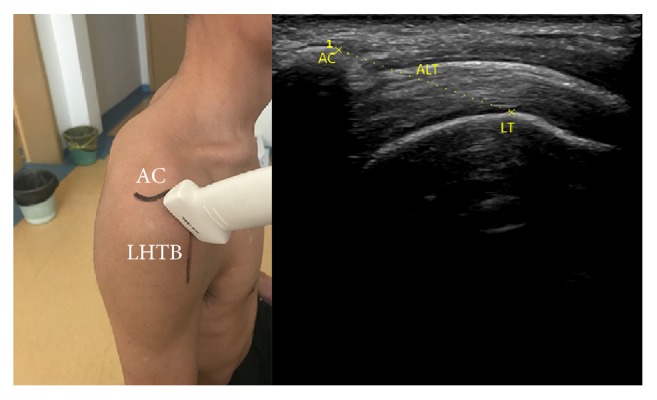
Anatomical structures; the position and orientation of transducer; and the ultrasound image: LT (lesser tuberosity) and ALT (acromion-lesser tuberosity).
